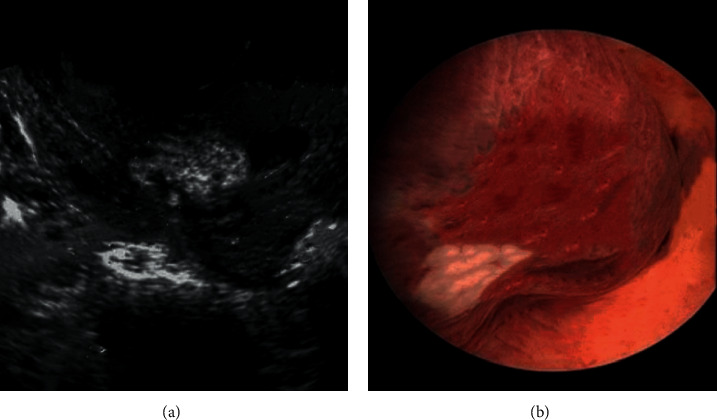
(a) Ultrasound image of uterine fetal residues; (b) hysteroscopy image of uterine fetal residues.
